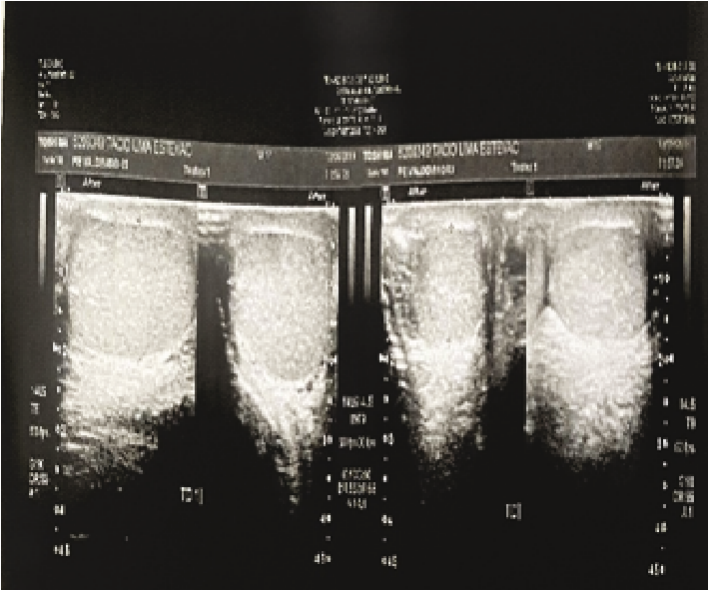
Ultrasound image of the scrotum.
